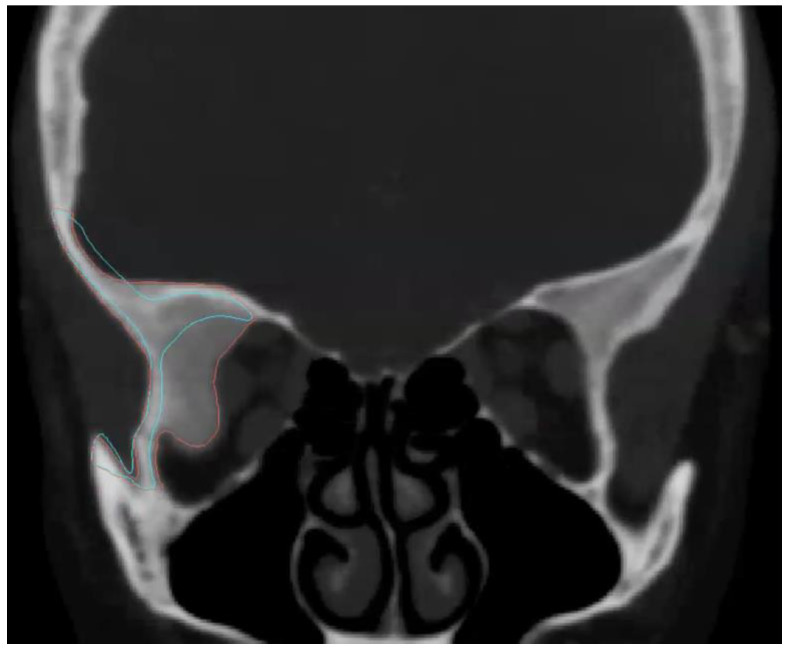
The preoperative CT scan of Patient 5 indicates a lesion consistent with meningioma in the fronto-orbital region (highlighted in red). The virtual design of the PEEK PSI is represented in blue.
